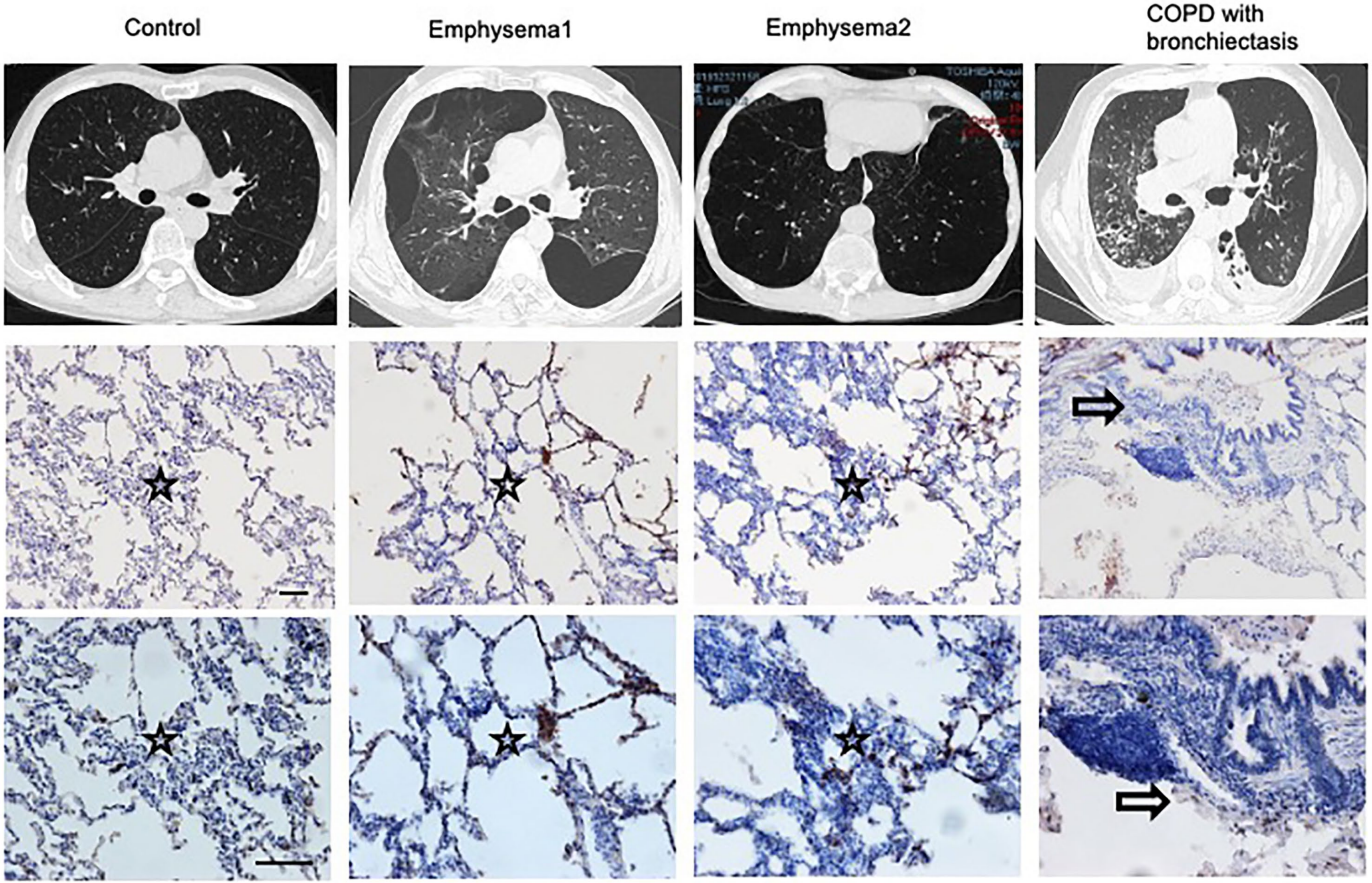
Heterogenicity of COPD patients. CT scans and lung slices from a donor (Control), two patients with emphysema, and one COPD patient with bronchiectasis who received lung transplantation. (Upper, × 100; Bottom, × 200), Bar: 100 μm. Hollow arrow: airway. Star: interstitia and alveoli.
